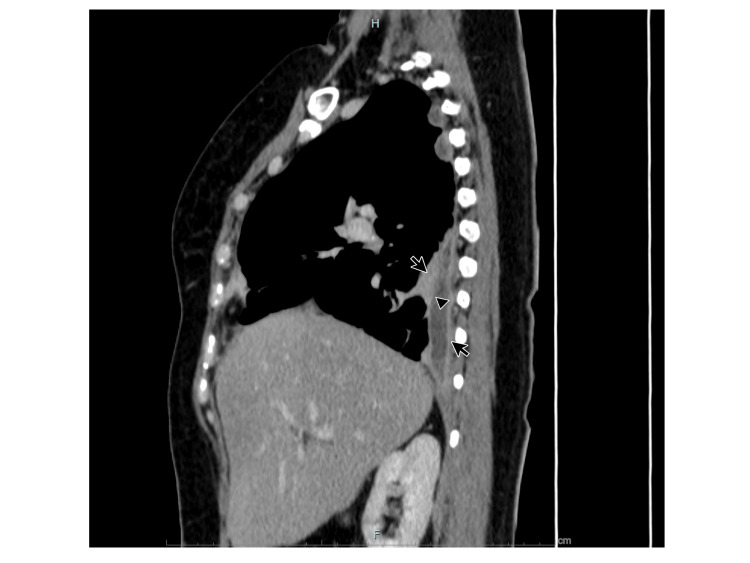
CT pulmonary sagittal view. Arrowhead: Heterogeneous hypodense material in the pleural space. Small arrows: Pleural thickening along with split sign.
